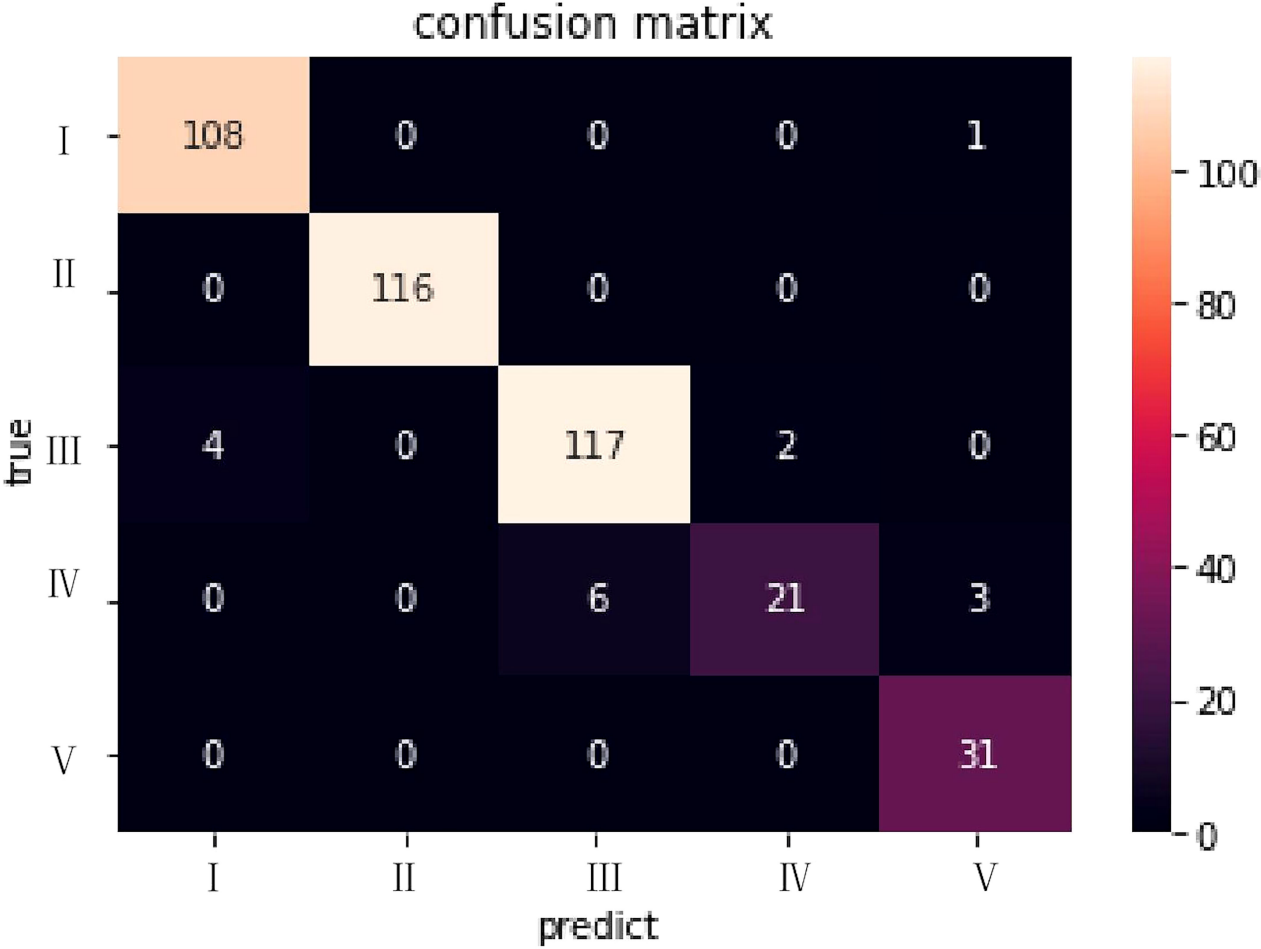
The confusion matrix for the XGBoost model in the dataset of CT with/without contrast. The abscissa represented the predicted results and the ordinate the actual results.
